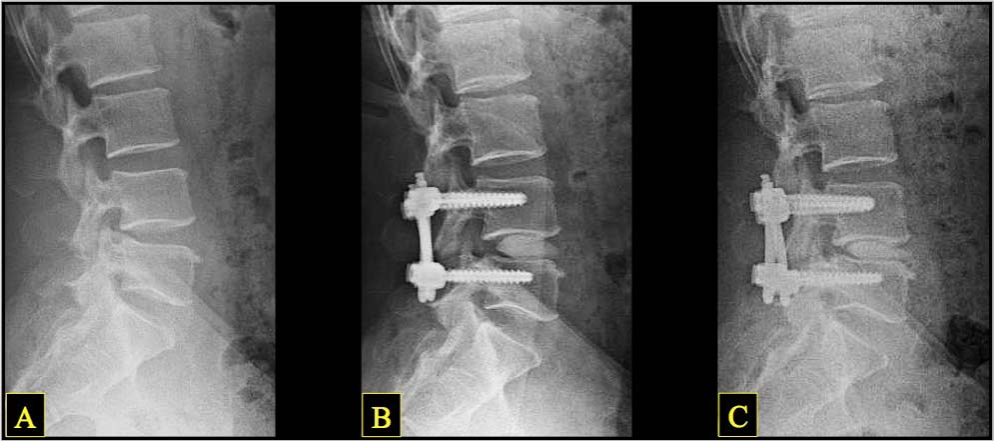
Representative lateral lumbar x-ray sequence in a single patient, showing preoperative (A), postoperative (B), and long-term follow-up (C) images. Note the changes in disc and neuroforaminal height: a marked increase from A to B, and slight decrease from B to C.
